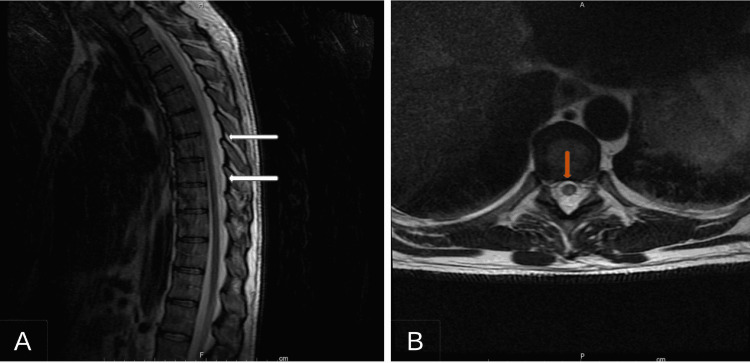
MR imaging of the thoracic spine T2/FLAIR sagittal view (A) Sagittal view of the thoracic spinal cord and (B) axial view of the thoracic spinal cord.
